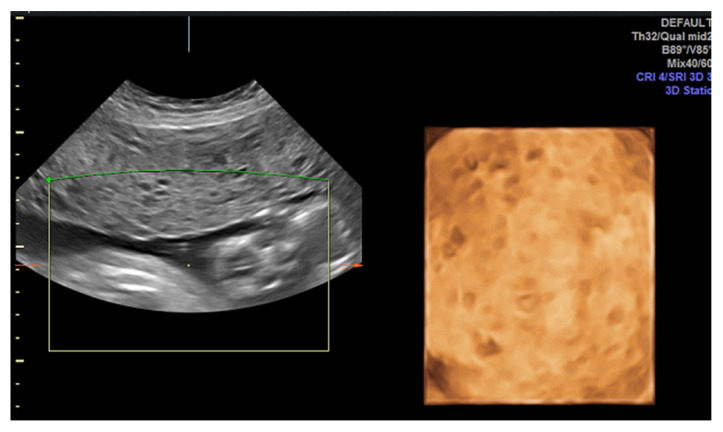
Ultrasound of partial hydatidiform mole. A nonviable fetus at 14 weeks’ gestation is visible, along with a placental region containing two large anechoic areas.
